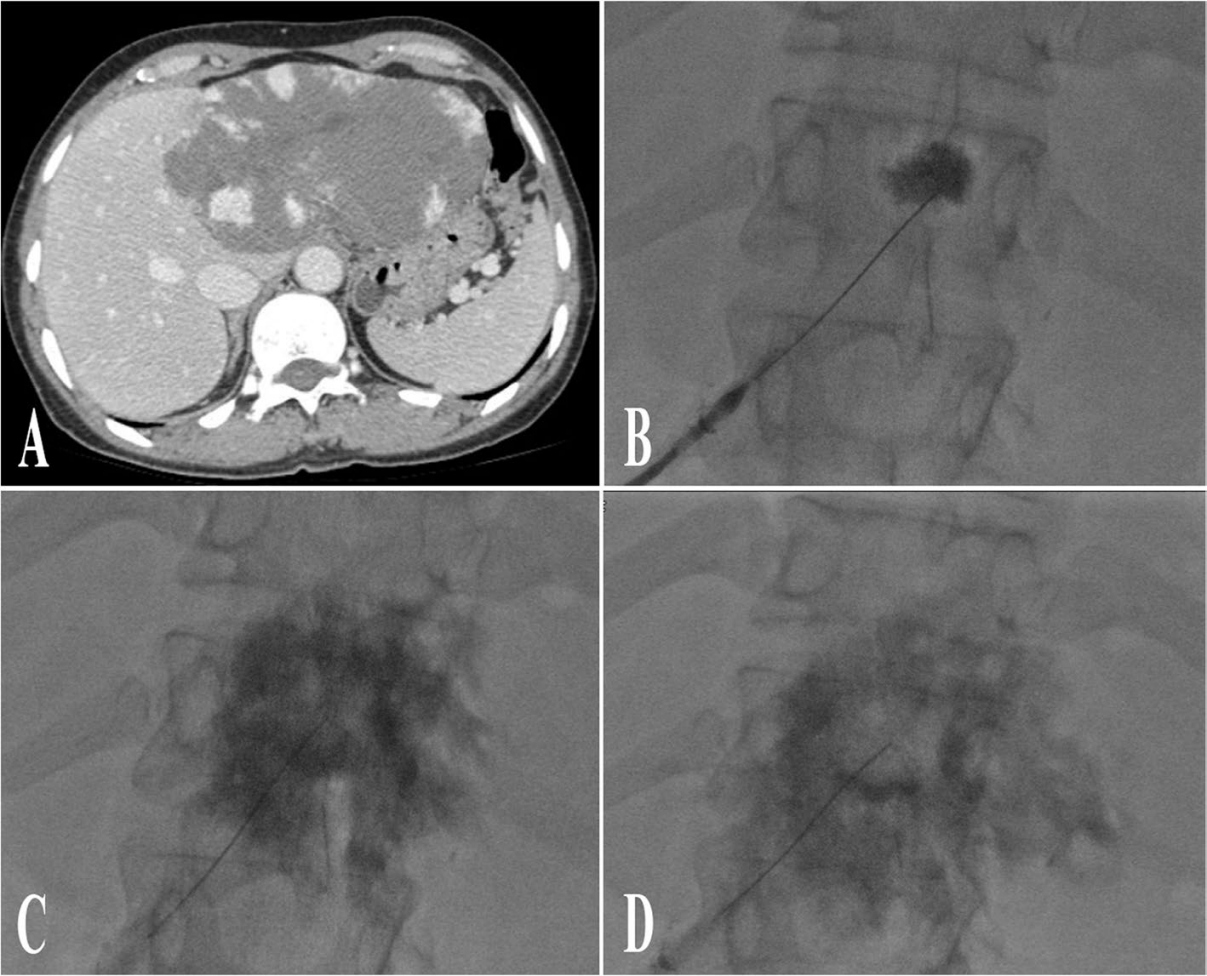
A Giant HVM with typical enhancement in the left liver lobe in a middle-aged woman complaining of chronic abdominal pain. B After ultrasound-guided access, contrast injection with fluoroscopic surveillance is performed to check the needle tip position. C, D Injection of bleomycin solution shows gradual rarefaction and dissemination of contrast
